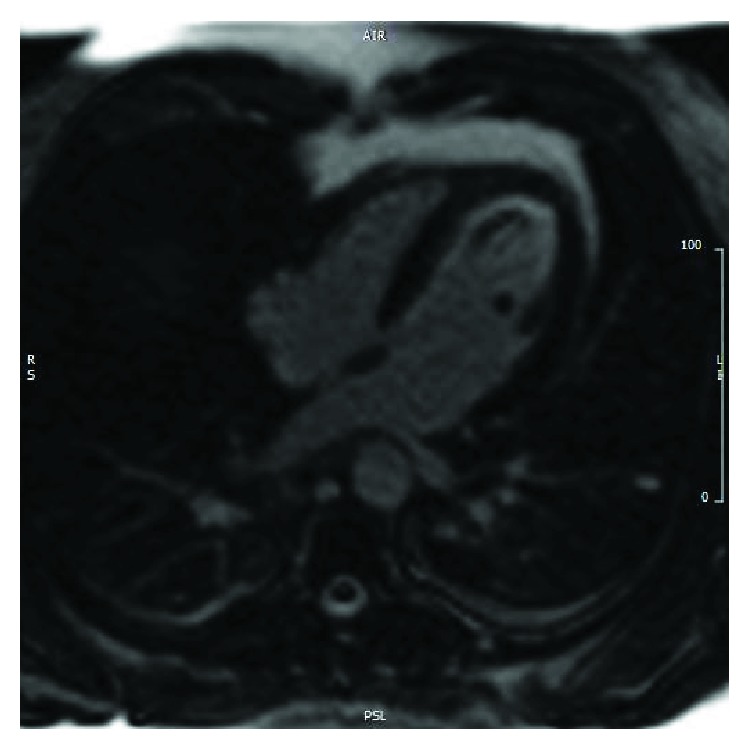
Cardiac magnetic resonance imaging 4-chamber-view post gadolinium injection revealing late gadolinium enhancement of the endomyocardium at the left ventricular apex.
